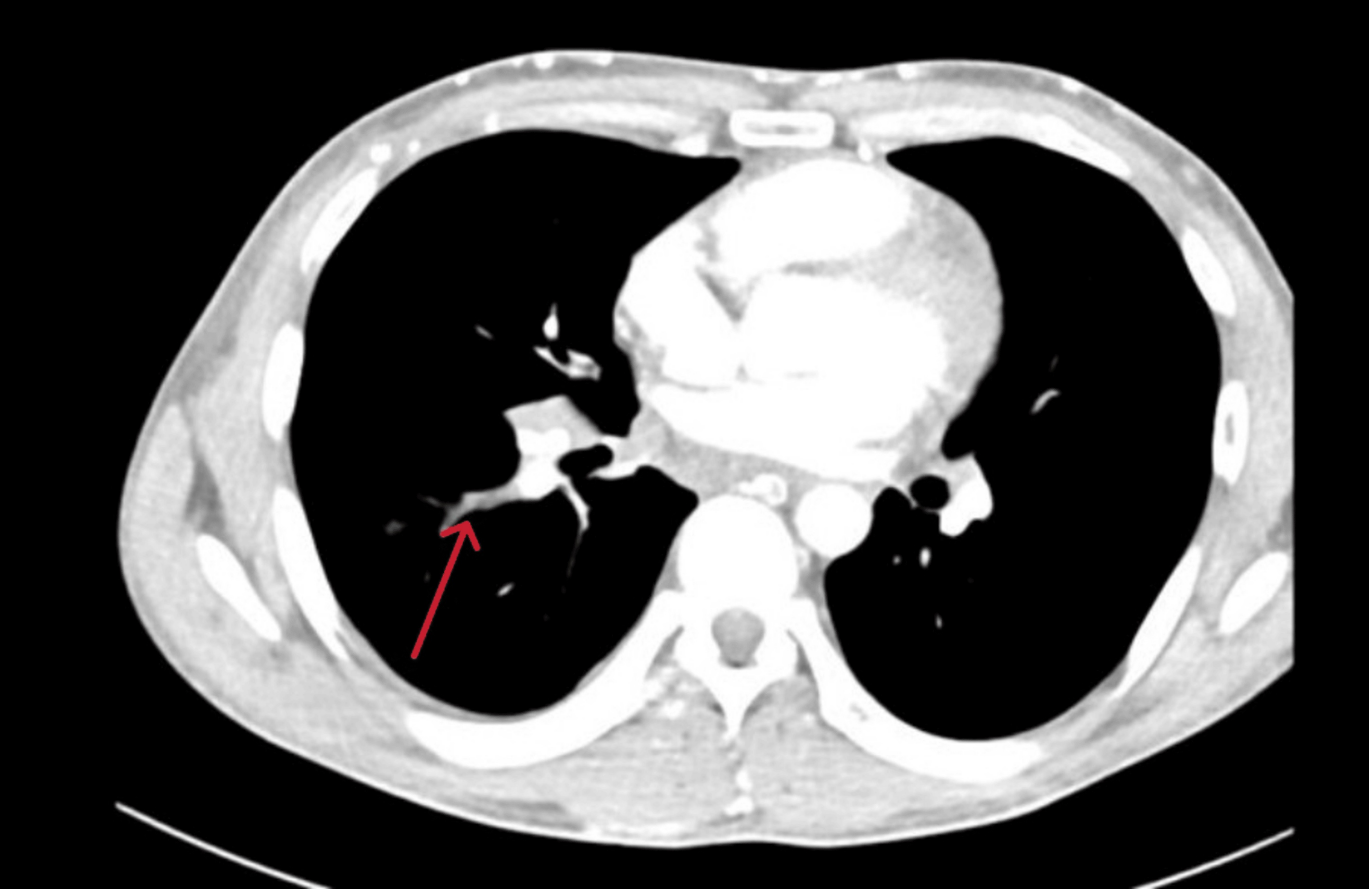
Computed tomography pulmonary angiography showing right-sided pulmonary emboli (red arrow)
